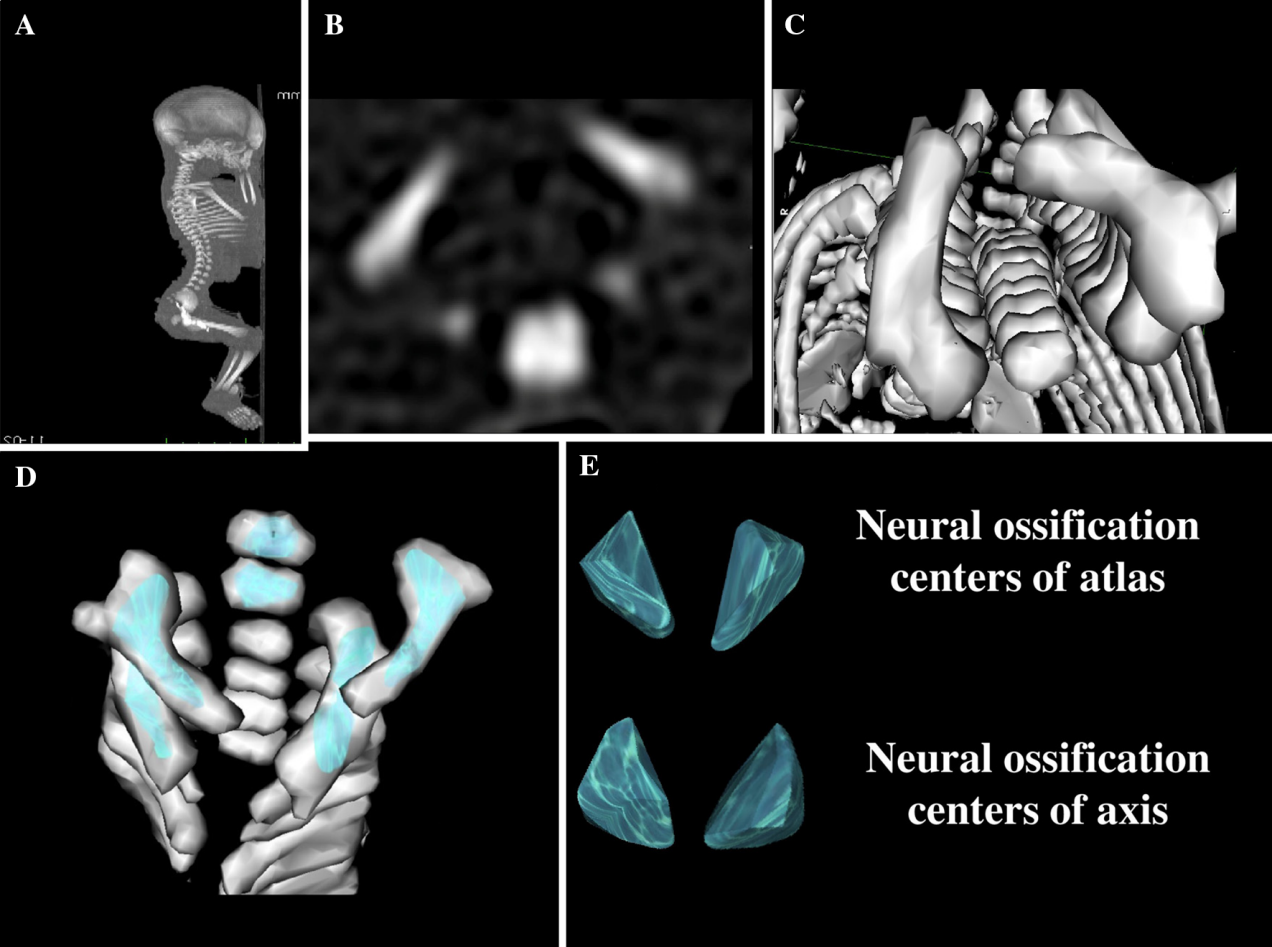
CT of a male fetus aged 22 weeks in the sagittal (a) and transverse (b) projections of the cervical vertebrae, reconstruction of the cervical vertebrae in the transverse projection (c), reconstruction of the atlas and axis using Osirix 3.9 (d), and neural ossification centers of the atlas and axis (e)
